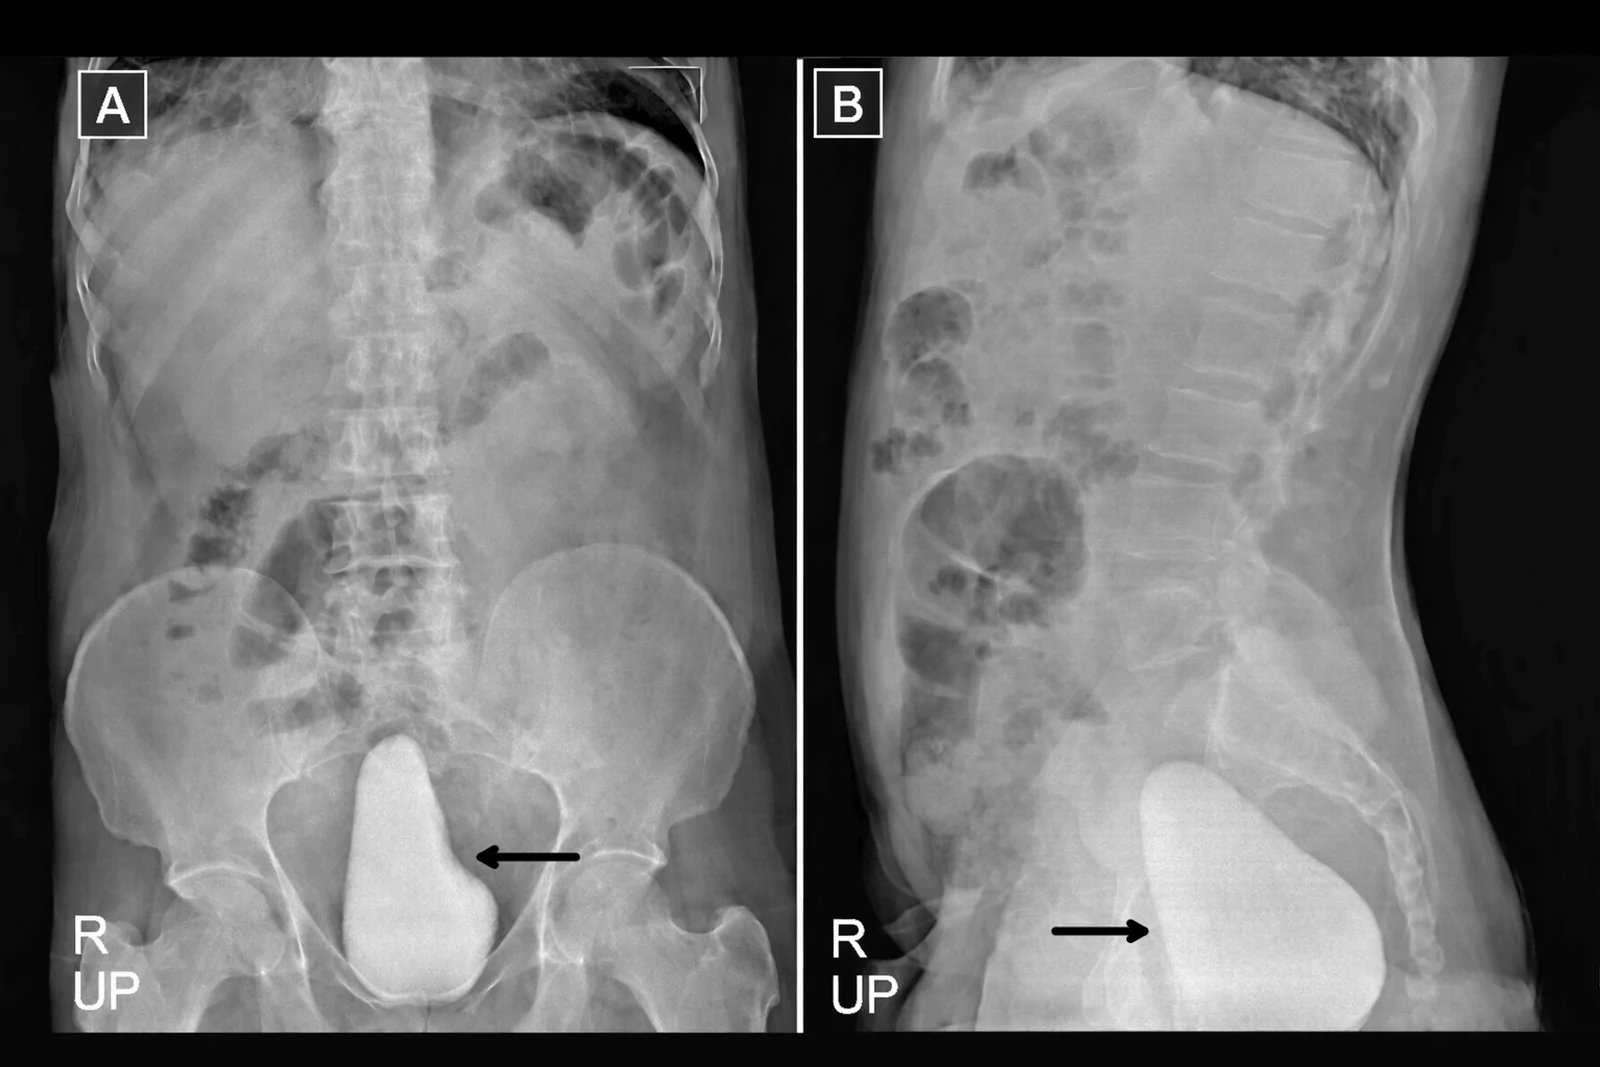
Plain abdominal radiographs showing a foreign body (arrows) impacted in the rectum. (A) Anteroposterior view. (B) Lateral view.
